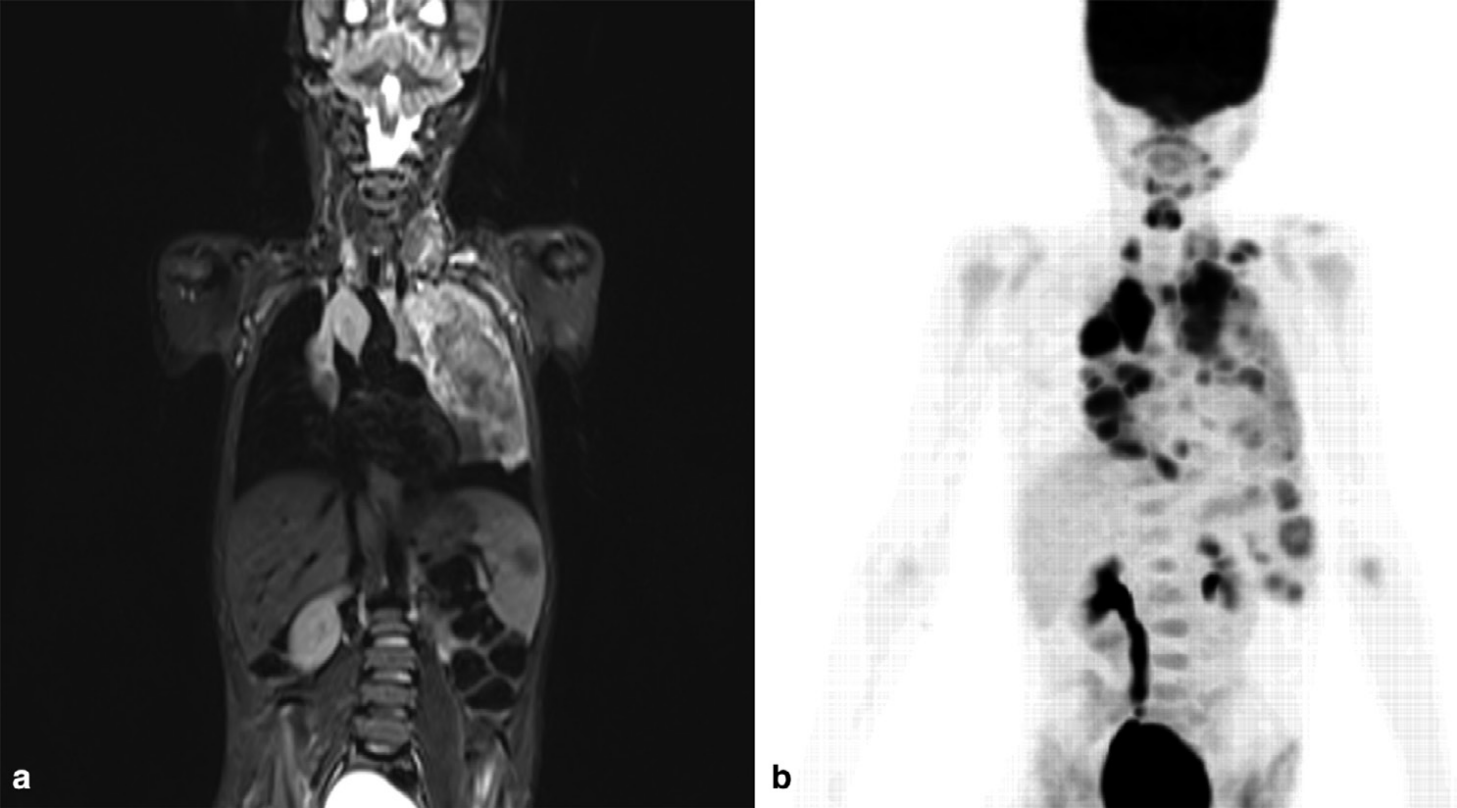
Coronal whole-body STIR MRI sequence (A) demonstrates gross mediastinal lymphadenopathy with left lung infiltration, and splenomegaly with a focal lymphomatous deposit in a 9-year-old boy with Hodgkin’s Lymphoma. (B) Whole-body maximum intensity projection image from the concomitant 18-FDG-PET/MRI examination demonstrates disease above and below the diaphragm and obstructive right hydronephrosis.
